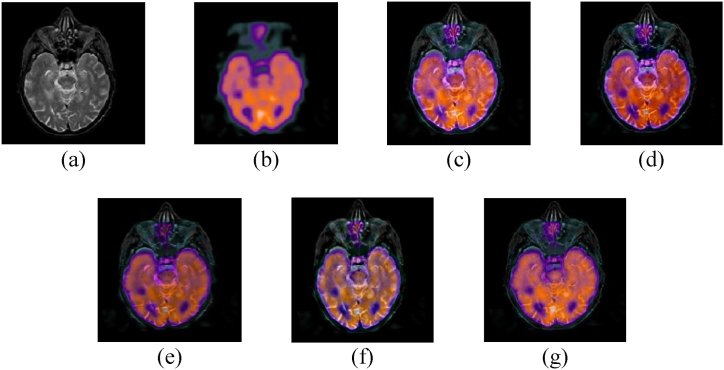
MRI and SPECT images fusion results of Normal aging, (a) MRI image, (b) PET image, (c) NSST-PAPCNN [24], (d) CNN [25], (e) CSMCA [26], (f) IFCNN [27], (g) Proposed.
